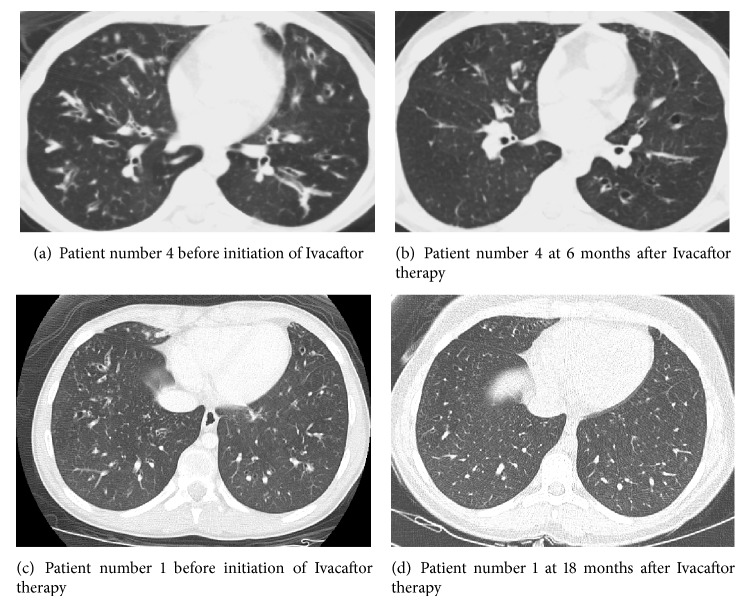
CT of the chest before and after Ivacaftor. Two representative chest CT slices of patient number 4 ((a) and (b), 16-year-old male) at the same anatomic region before and after Ivacaftor therapy. They demonstrate decreased mucus impaction in the latter scan despite the continued presence of bronchiectasis. Decreased mucus impaction probably explains improved lung function (Figures 2 and 3) despite irreversible nature of bronchiectasis. In the bottom panels ((c) and (d)) are the chest CT of another patient (14-year-old female) who already had established bronchiectasis in the peripheral airways before Ivacaftor (c) which has been resolved 18 months after Ivacaftor therapy (d).
